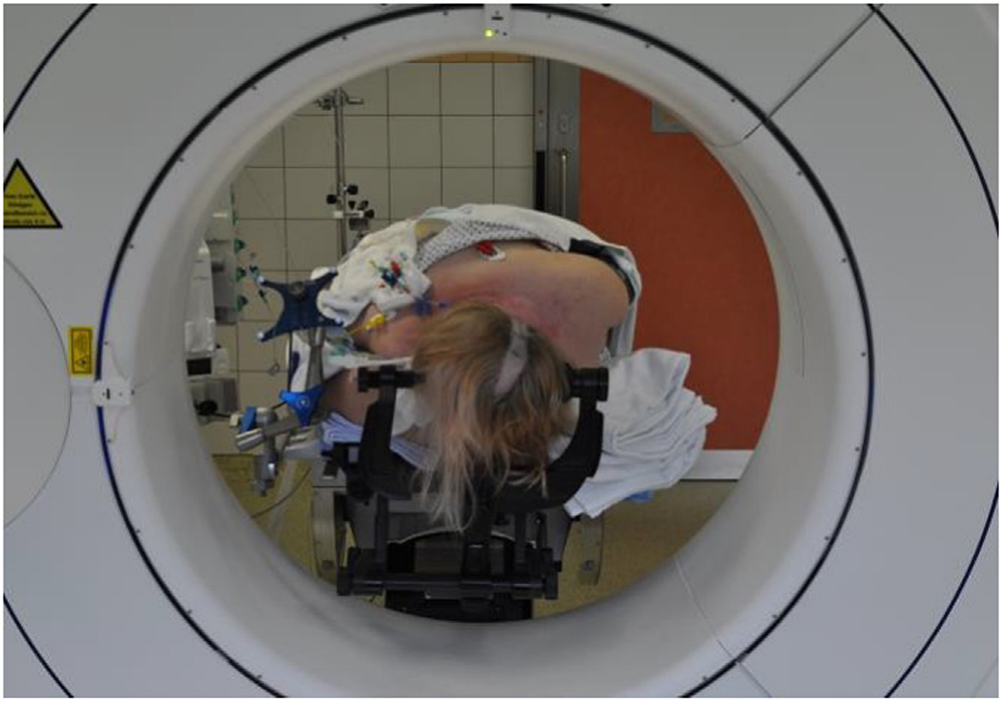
Carbon head mount and neuronavigation stereotactic reference attached to an additional mount. Placement of the patient in the cone beam computed tomography bore.
